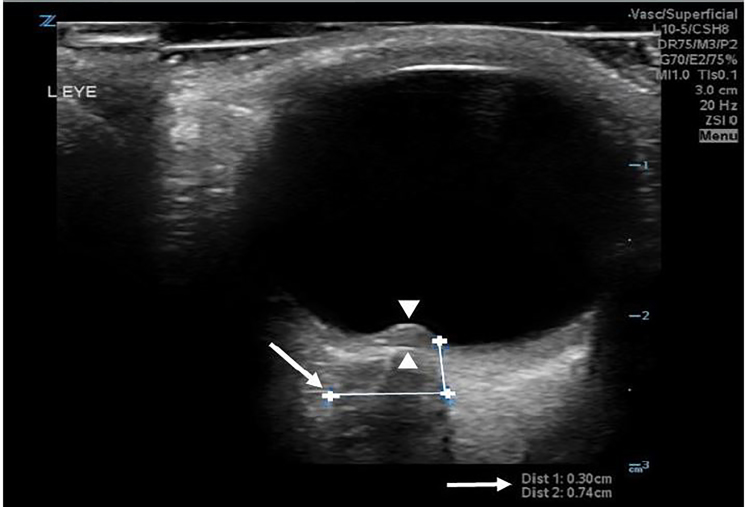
Left ocular ultrasound demonstrating optic disc elevation into the vitreous cavity (white arrow heads), as well as optic nerve sheath diameter measurements (white arrows)
